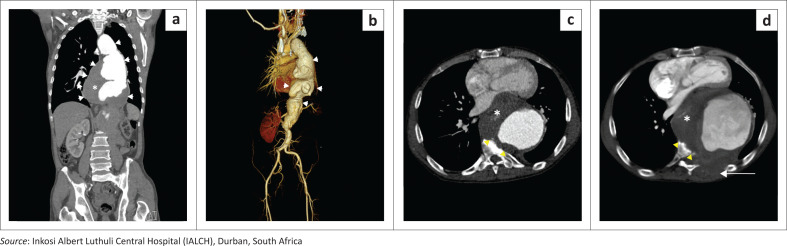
A 48-year-old man with a 3-month history of abdominal and back pain referred to the vascular unit. (a) Coronal CT angiography shows a large, multilobulated fusiform thoracoabdominal aortic aneurysm (*white arrowheads*). (b) 3D volume-rendered CT image demonstrates the fusiform aneurysm extent (*white arrowheads*). (c) Axial CTA shows anterior vertebral erosions (yellow arrowheads). (d) Follow-up CTA 1 month later shows progression of the erosions (*yellow arrowheads*) and extension of the haematoma into the posterior chest wall (*white arrow*). Eccentric intraluminal thrombus is noted (*). Surgery was not performed owing to extensive disease.
